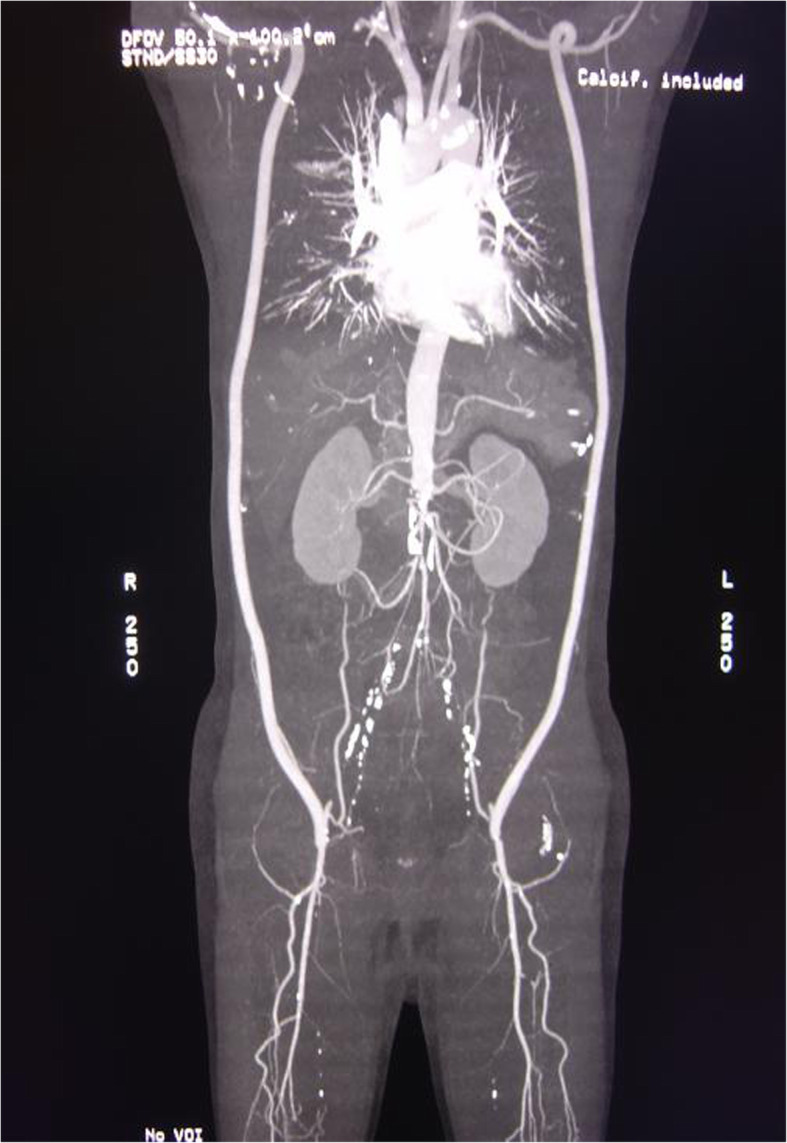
The patients previously underwent bilateral axillofemoral bypass due to Leriche syndrome. Postoperative CTA examination shows occlusion of the infrarenal abdominal aorta, bilateral internal iliac artery, external iliac artery, and bilateral superficial femoral artery. The deep femoral artery is well compensated, and the bilateral collateral circulation is unobstructed
